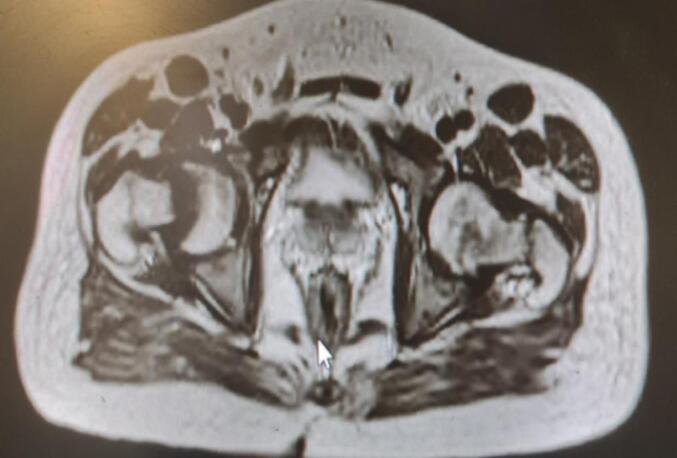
MRI of Bilateral femoral neck fracture with slipped right femoral head and AVN in right femoral.
